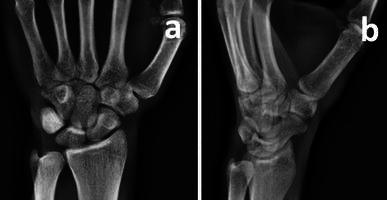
Final hand radiographs 6 months after injury
